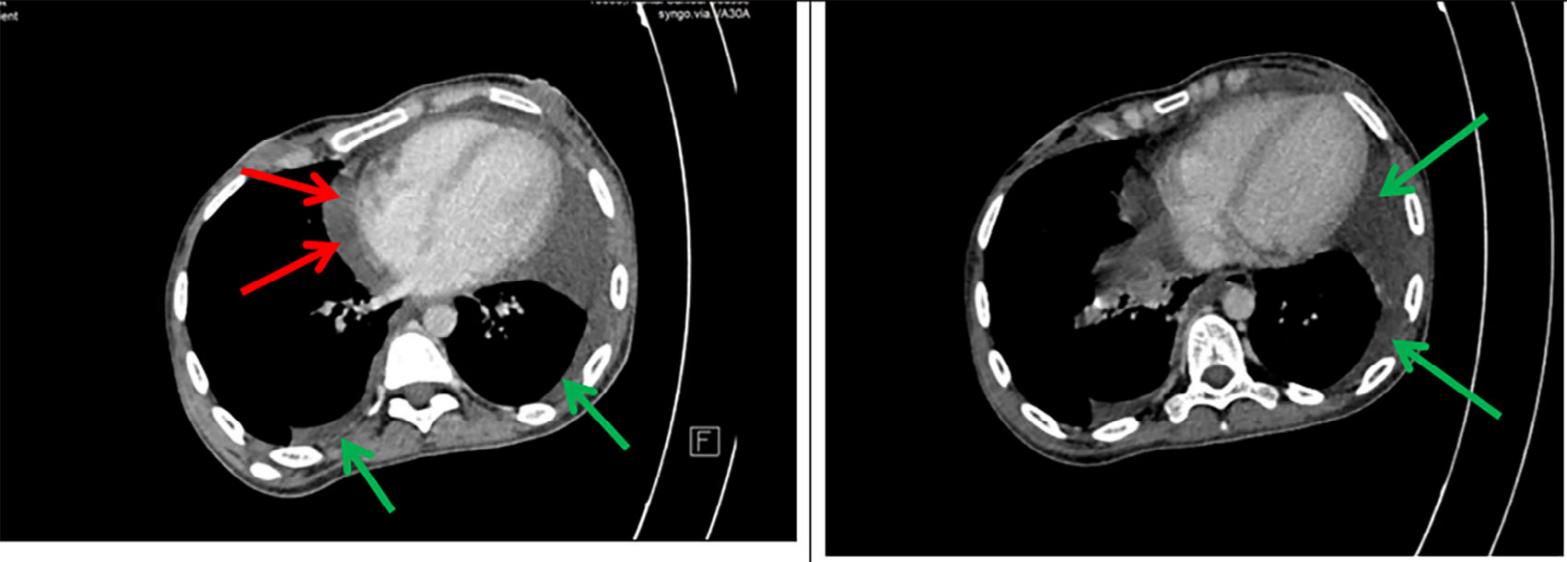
Axial contrast-enhanced CT scan of the chest shows bilateral low abundance pleural effusion (green arrows) and low abundance pericardial effusion (red arrows).
